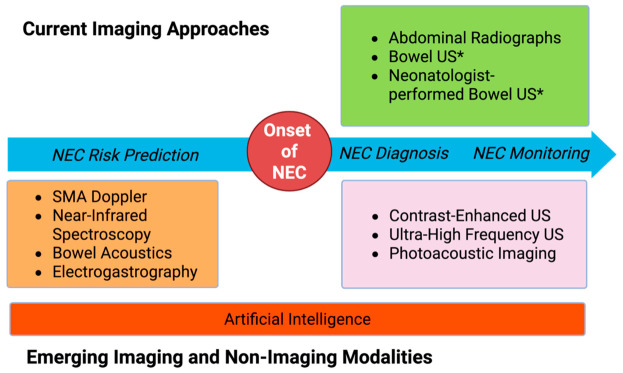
Current and Emerging Approaches to NEC Evaluation. Current imaging strategies (green box) for NEC primarily begin after clinical onset and are used for disease diagnosis and monitoring. Abdominal radiography remains the most widely used initial modality, while bowel ultrasound can provide additional physiologic information when available (*), although access and expertise vary across institutions. Emerging imaging modalities (purple box) may further expand the ability to characterize intestinal injury and support both diagnosis and monitoring. In addition, physiologic monitoring approaches (orange box) aim to detect early alterations in intestinal perfusion, oxygenation, or motility and therefore hold potential for NEC risk prediction before overt clinical disease develops. Artificial intelligence (red box) may further enhance these approaches by integrating imaging and physiologic data to improve risk prediction, diagnostic interpretation, and clinical decision-making.
